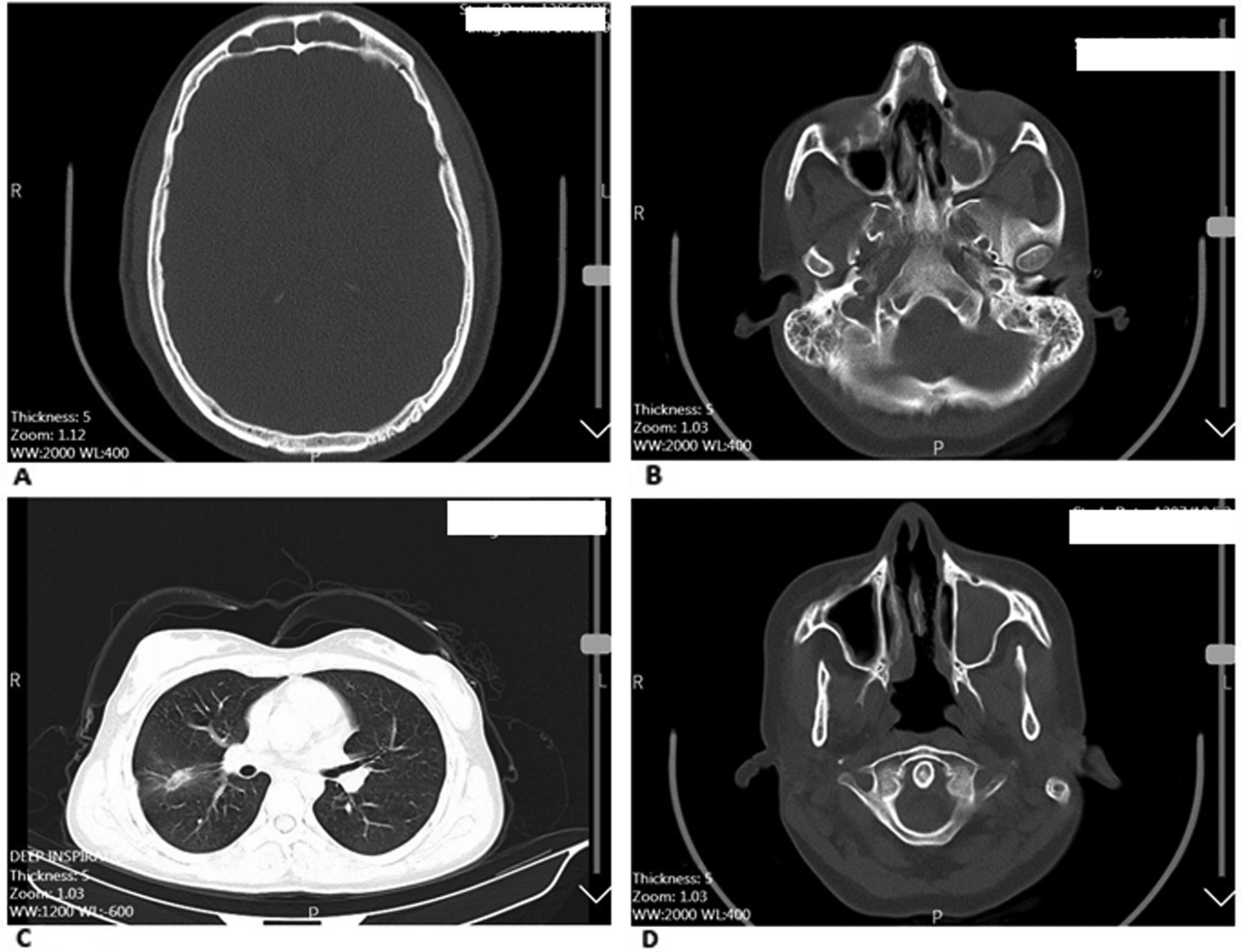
Radiographs of a Wegener granulomatosis patient: (
